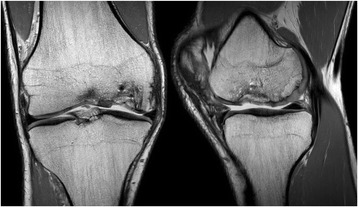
Pre-Treatment Proton Density (PD) weighted Coronal and Sagittal MRI images of the knee showing the isolated chondral defect involving the central weight bearing area of the medial femoral condyle
